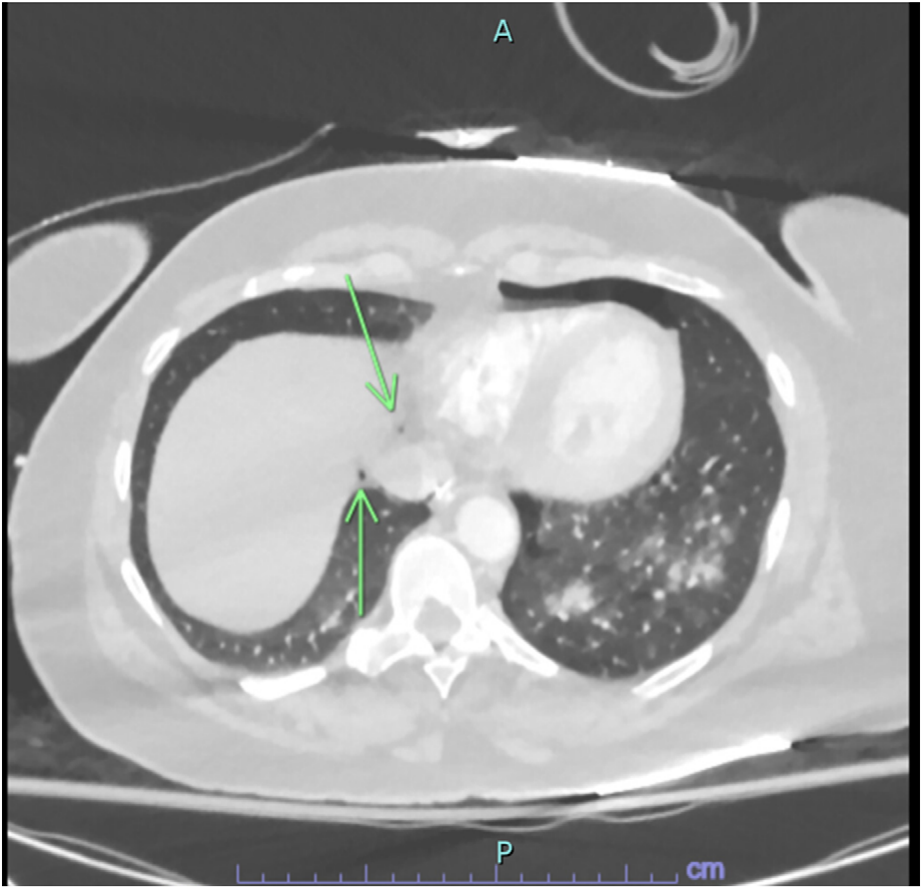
Initial computed tomography with pulmonary embolism protocol performed, revealing pneumomediastinum in the lower portion of the mediastinum (green arrows) favored to be secondary to alveolar rupture as well as a left-sided pneumothorax.
